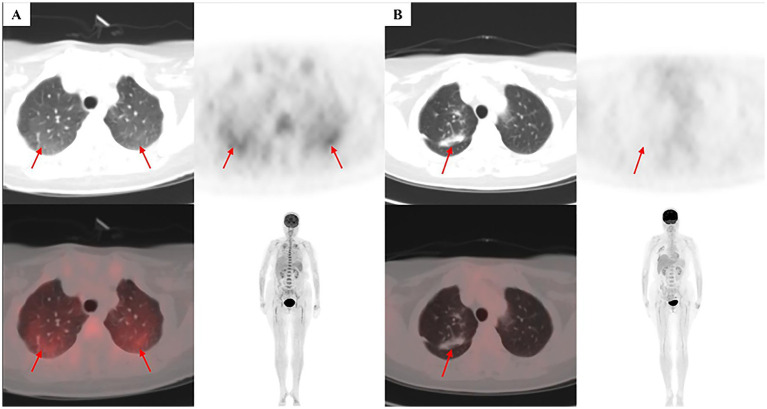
Positron emission tomography-computed tomography (PET-CT) findings at presentation and after treatment. (A) Initial scan (July 19, 2025) reveals diffuse, bilateral pulmonary fluorodeoxyglucose (FDG) uptake, most pronounced in the upper lobes (arrows), with a maximum standardized uptake value (SUVmax) of 3.0 in the upper lung fields versus 1.0 in the remaining parenchyma. (B) Follow-up scan (October 14, 2025) after systemic immunochemotherapy demonstrates complete resolution of the previously observed hypermetabolic foci, consistent with a complete metabolic response (CMR). The linear opacity in the right upper lobe (arrowhead) is compatible with post-surgical change following the diagnostic wedge resection.
